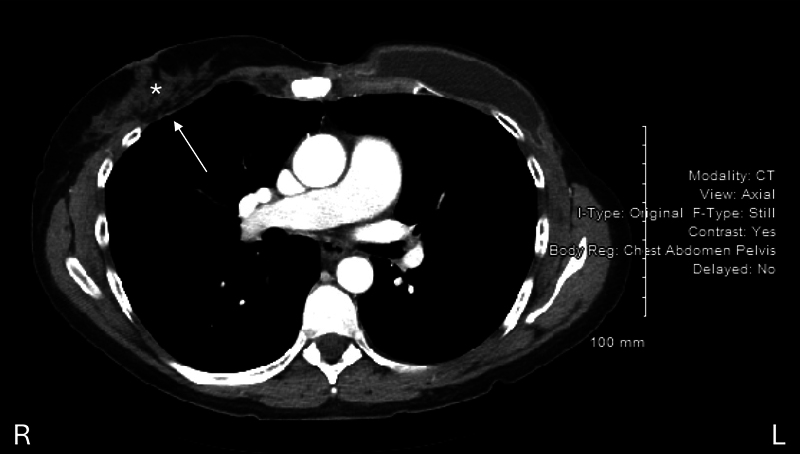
CT imaging demonstrating absence of the right prosthesis (*) with changes to the chest wall arrow).
